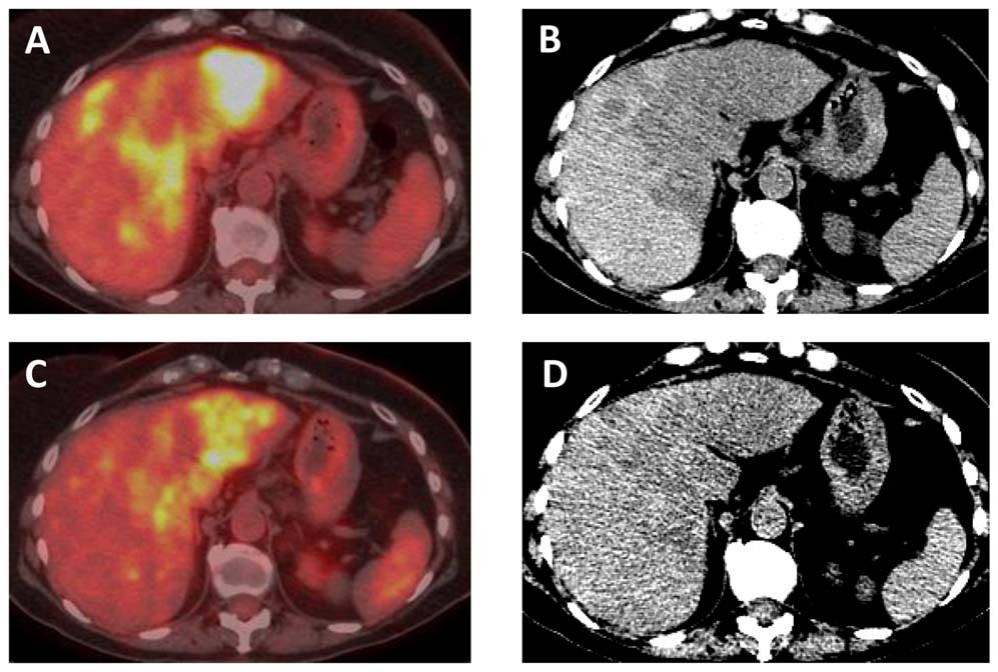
A 68 year old female with *KRAS wt* intrahepatic CCA. Axial (A) fused PET-CT and (B) unenhanced CT images demonstrate multiple confluent FDG avid liver metastases. After 3 months of erlotinib therapy, (C) axial fused PET-CT and (D) unenhanced CT images show decreased FDG avidity and slightly decreased size of metastases.
